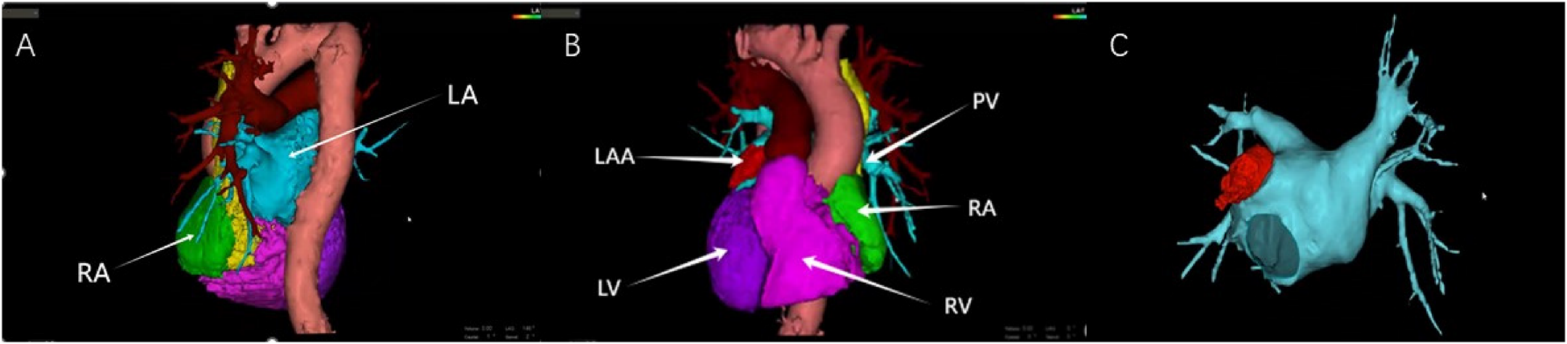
(A) Computed tomography (CT) three-dimensional (3D) reconstruction of the heart (RA, right atrium; LA, left atrium); (B) CT 3D reconstruction of the heart (LAA, left atrial appendage, LV, left ventricle, PV, pulmonary vein, RA, right atrium, and RV, right ventricle); (C) CT 3D reconstruction of the left atrium.
